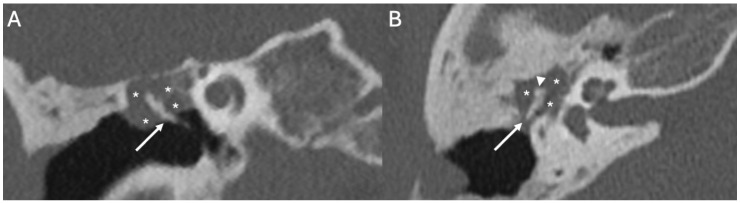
Menigitis due to *Streptococcus pneumoniae* in a 19-year-old patient with recurrent acquired Cholesteatoma. Coronal reformat (A) and axial CT (B) show hypodense material in the middle ear (epitympanum and mesotympanum, asterisks in (A,B)), with erosion of the incus (arrows in (A,B)), and malleus (arrowhead in (B)).
